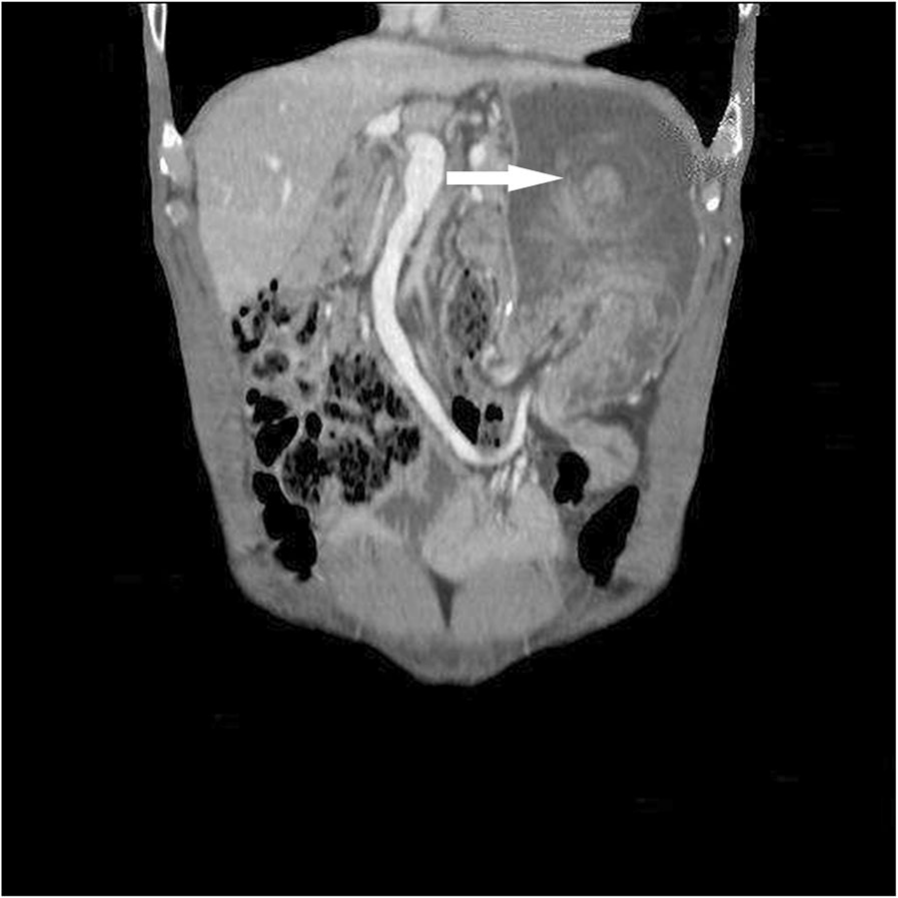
Abdominal CT scan. Another section showed loop of jejunum in the body of stomach (Arrow: jejunogastric intussusception)
